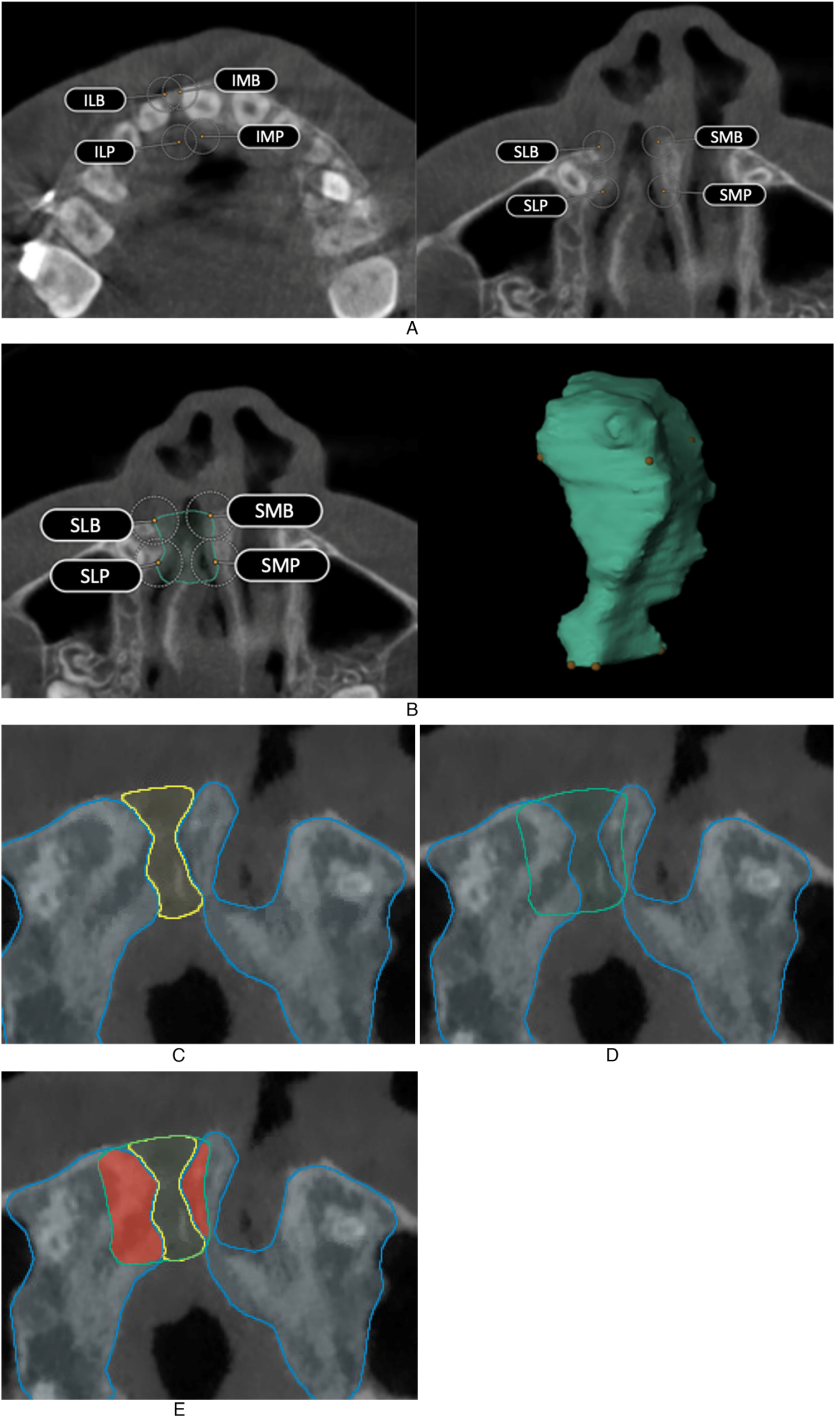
Cleft volume calculation process. (A) Landmark placement in the preoperative CBCT
